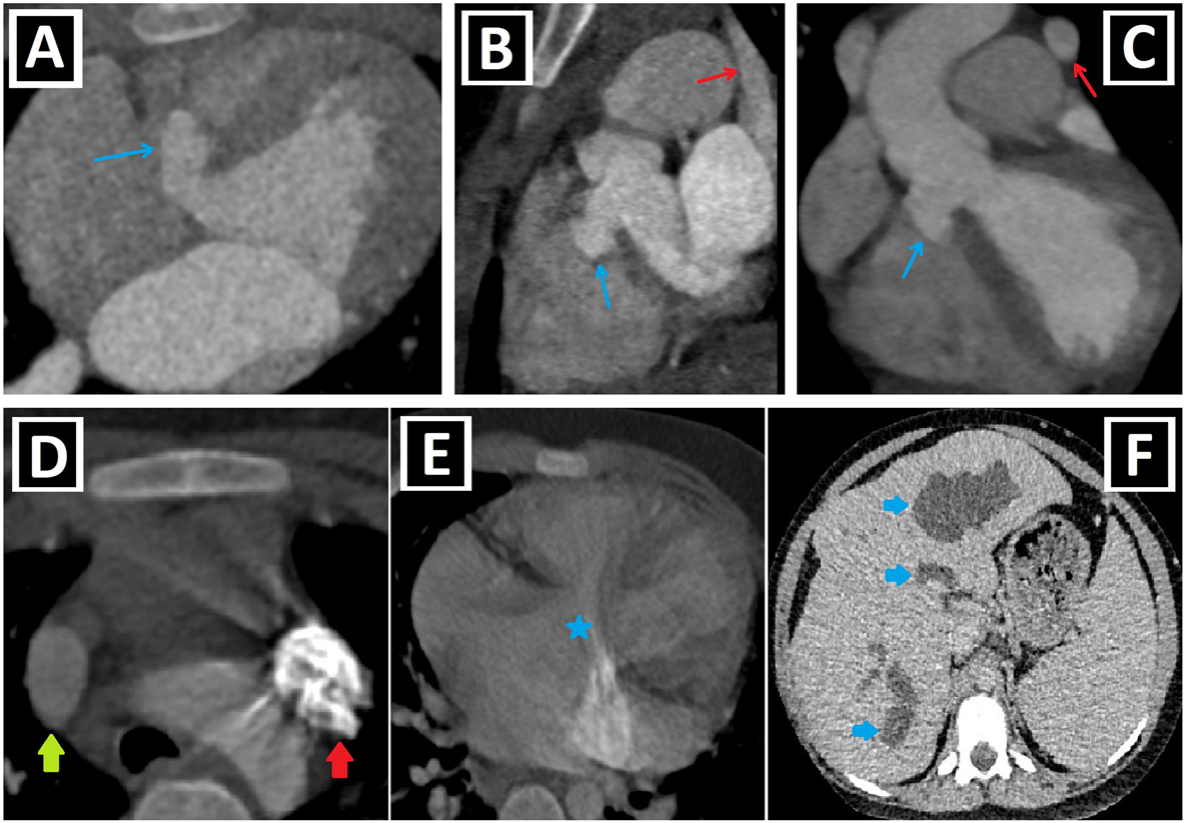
Shunt lesions accompanying PLSVC-2. a–c Axial (a), oblique sagittal (b), and oblique coronal (c) CT images depict interventricular membranous septal aneurysm (blue arrows) developing due to the spontaneous closure of VSD in a patient with PLSVC (red arrows). d–f Axial CT images indicate accompanying AVSD (blue star) in a patient with Caroli disease (blue arrows showing multifocal cystic dilatation of segmental intrahepatic bile ducts) and double SVC (green and red arrows).
